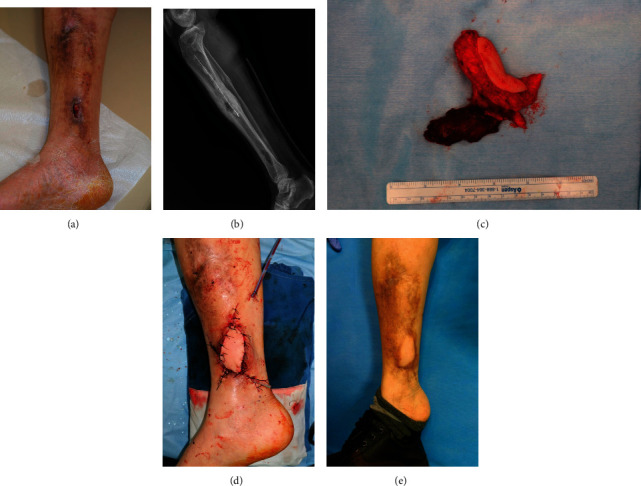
(a) A 58-year-old male with persistent wound problem on right distal leg. (b) Preoperative lower leg X-ray showed osteolytic lesion on tibia. (c) Harvested lateral arm free flap. (d) Immediate postoperative image. (e) Postoperative 10 months.
